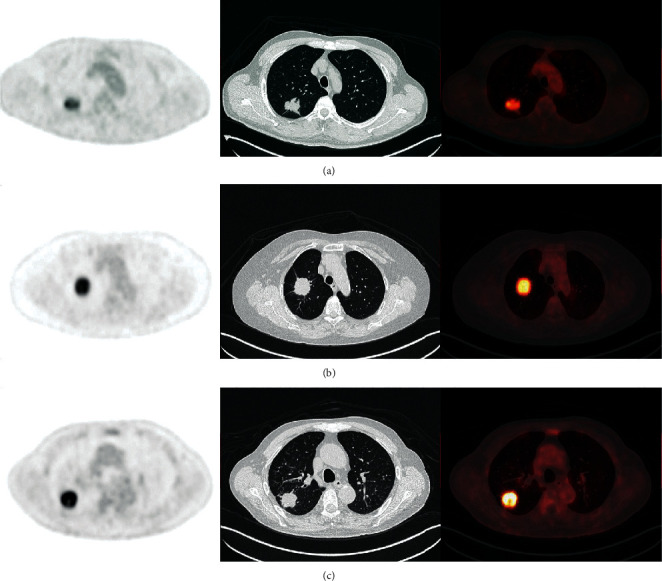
Representative images of ^18^F-fluorodeoxyglucose positron emission tomography/computed tomography (PET/CT), showing variations in the maximum standardized uptake (SUVmax) value in relation to the expression level of programmed cell death ligand-1 (PD-L1). (a) PET/CT images showing FDG uptake by PD-L1-negative adenocarcinoma (ADC) (SUVmax 6.91, size 3.0 cm, and PD-L1 expression < 1%). (b) PET/CT images showing FDG uptake by ADC with low PD-L1 expression (SUVmax 11.37, size 3.1 cm, and PD-L1 expression 1–49%). (c) PET/CT images showing intense FDG uptake by ADC with high PD-L1 expression (SUVmax 19.49, size 3.0 cm, and PD-L1 expression > 50%).
